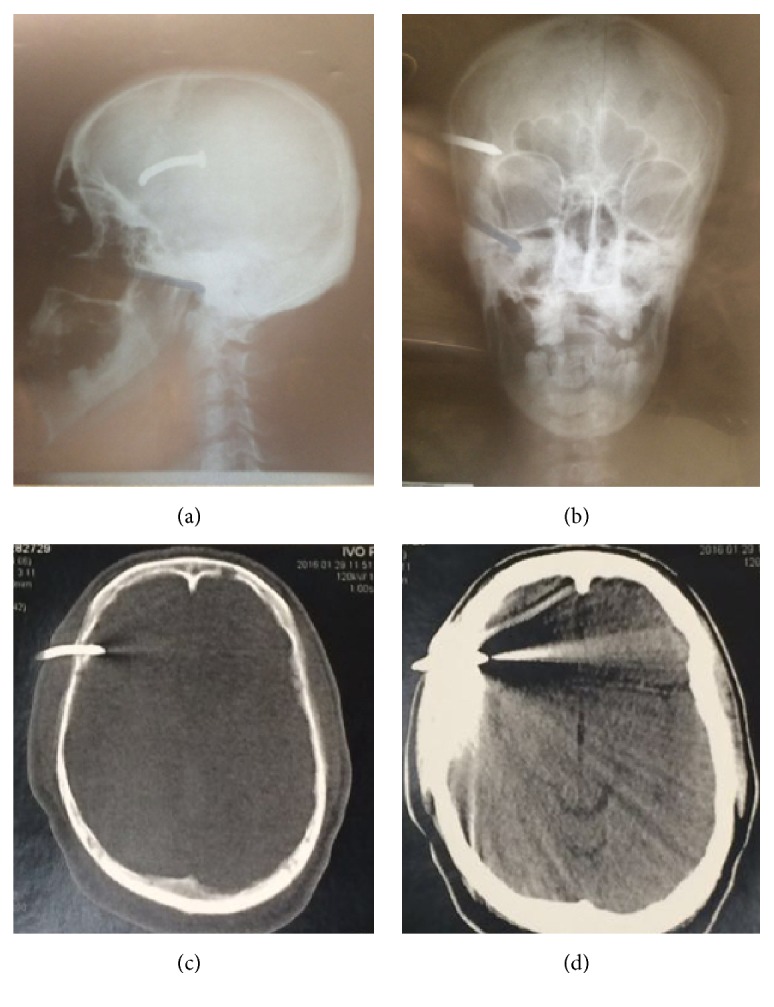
X-ray and head CT showing the presence of a nail on the frontotemporal region. (a) Preoperative simple sagittal skull X-ray showing a curvilinear, 7 cm long, oblique oriented nail in the skull. (b) Preoperative simple coronal skull X-ray showing a nail piercing the skull on the right frontotemporal region. (c) Axial head CT (bone window) showing one radiopaque nail which has penetrated both tables of skull and entered the brain parenchyma. (d) Axial head CT (soft tissue window) showing one radiopaque nail which has penetrated both tables of skull and entered the brain parenchyma.
